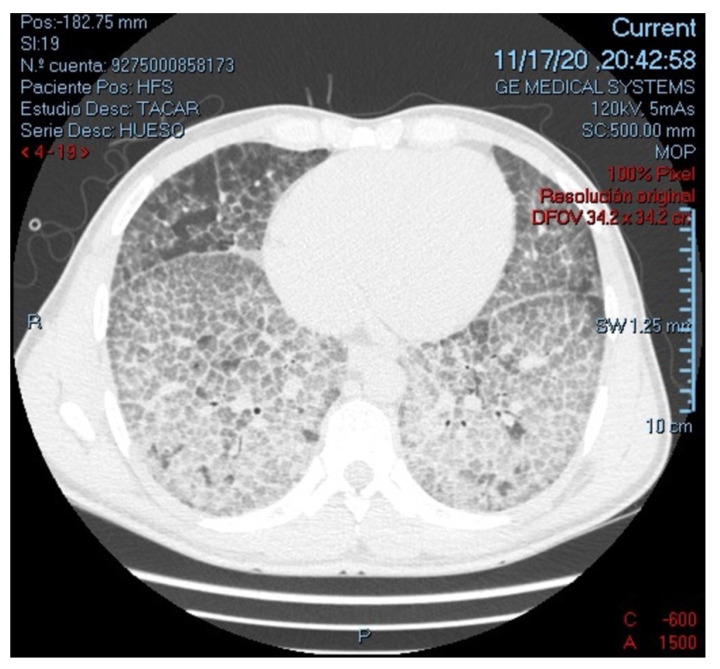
High-resolution computed tomography of the chest (parenchymal view), showing multiple ground-glass opacities with thickening of interlobular septae, configuring an extensive characteristic “Crazy-paving” pattern in both lungs.
